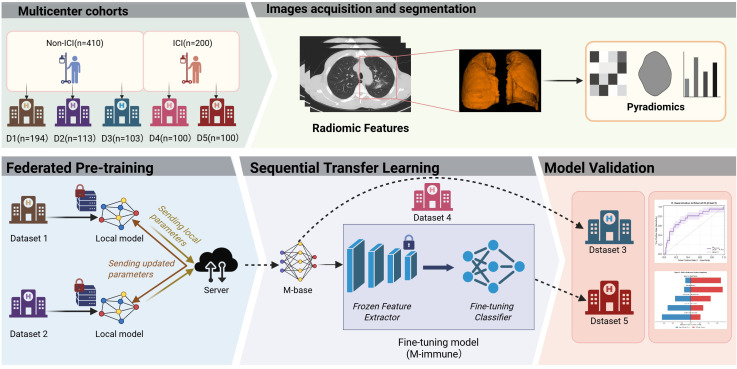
Study design. Multicenter cohorts were collected and divided into non-ICI and ICI populations. Chest CT images were acquired, lung regions were segmented, and radiomic features were extracted using PyRadiomics. A federated learning (FL) strategy was applied to pretrain a baseline model (M-base) on non-ICI cohorts (Dataset 1 and Dataset 2) without sharing raw data. The pretrained model was subsequently transferred to the ICI cohort (Dataset 4) via sequential transfer learning with a frozen feature extractor and fine-tuned classifier, resulting in an immune-specific model (M-immune). Model performance was evaluated on independent datasets (Dataset 3 and Dataset 5). ICI, immune checkpoint inhibitor; Non-ICI, patients who did not receive immune checkpoint inhibitor therapy; D1–D5, Dataset 1 to Dataset 5; CT, computed tomography; FL, federated learning; M-base, baseline model pretrained on non-ICI cohorts; M-immune, fine-tuned model for ICI-treated patients.
